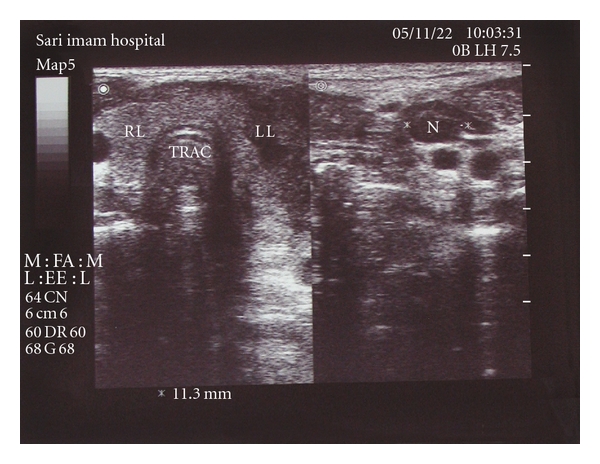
First sonography in the second case.
